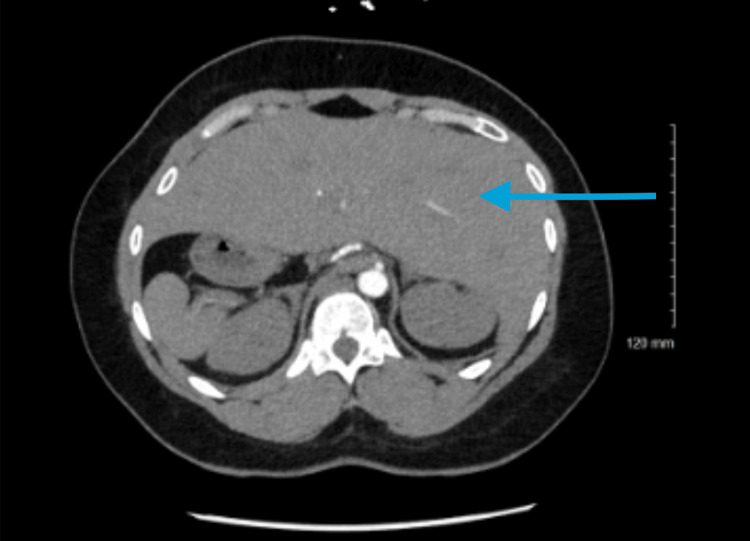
Axial contrast-enhanced CT demonstrates classic features of situs ambiguous with polysplenia. Multiple splenules are visualized along the right upper quadrant, consistent with right-sided polysplenia. The liver is predominantly left-sided, occupying most of the left hemiabdomen.
